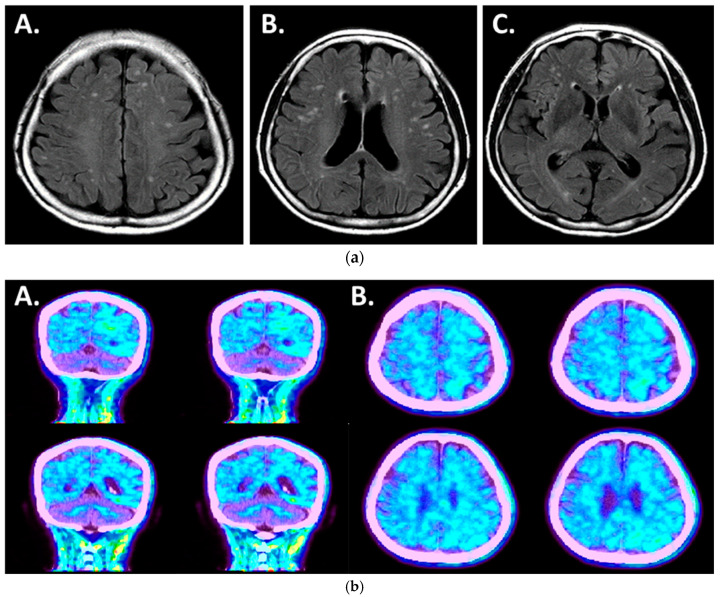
(a) Magnetic resonance imaging of the patient: observations of Axial FLAIR (A), (B), (C) sequences of the patient with mild diffuse brain atrophy. (b) Amyloid PET image of the patient: abnormal amyloid deposits observed in gray matter of whole brain, especially in the left temporal lobe. (A) Coronal plane. (B) Axial plane.
